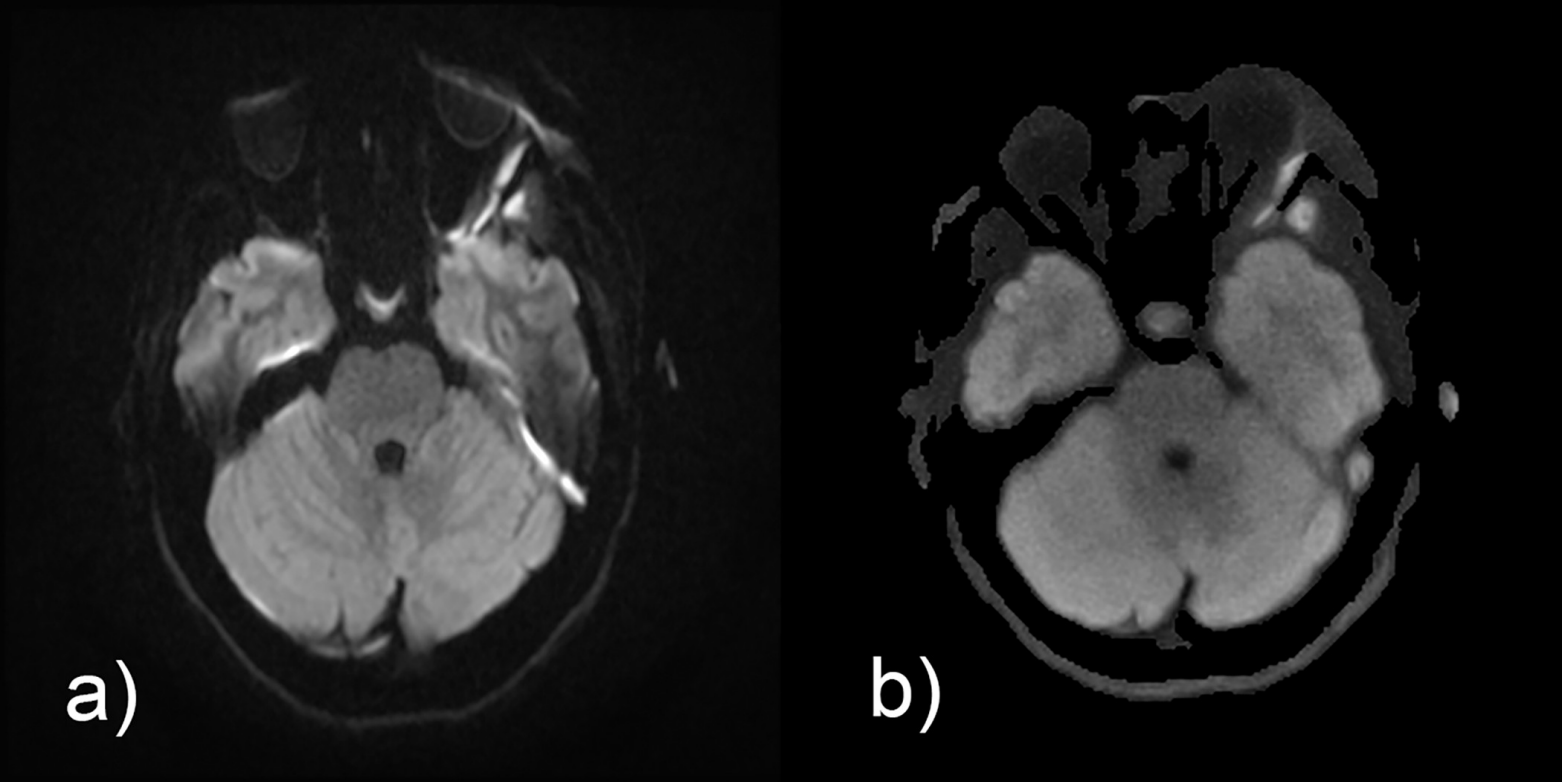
Comparison of isoDW images obtained by (a) EPI-DWI and (b) STEAM-DWI in a 13-year-old girl with left-sided postseptal inflammation and abscess lateral to the rectus lateralis muscle into the temporalis muscle. The diffusion restriction can be seen in both EPI-DWI and STEAM-DWI, but the morphological distortions and artificially increased signal at the anterior and posterior border of the conus make it difficult to accurately assess the extent of the abscess in EPI-DWI.
